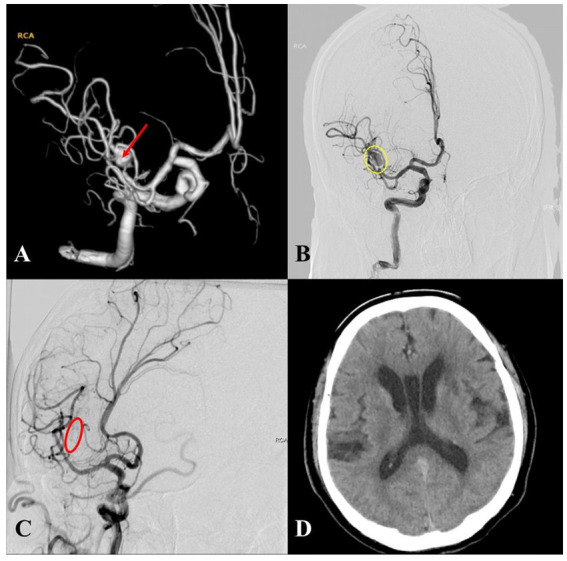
A case of postoperative chronic parent vessel occlusion after PED implantation. (A) Intraoperative 3D angiography revealed a non-saccular IA in the M3 segment of the right middle cerebral artery (red arrow). (B) A 3.00 × 20 mm PED Flex was placed in the right M2–M3 segment, marked remnant of contrast within the IA was observed in the immediate postoperative angiography (yellow circle). (C) One-year postoperative follow-up DSA revealed occlusion of the parent vessel (red circle), with the patient reporting no discomfort after intervention. (D) One-year follow-up head CT scan showed no evidence of cerebral infarction at the site of the occluded artery.
